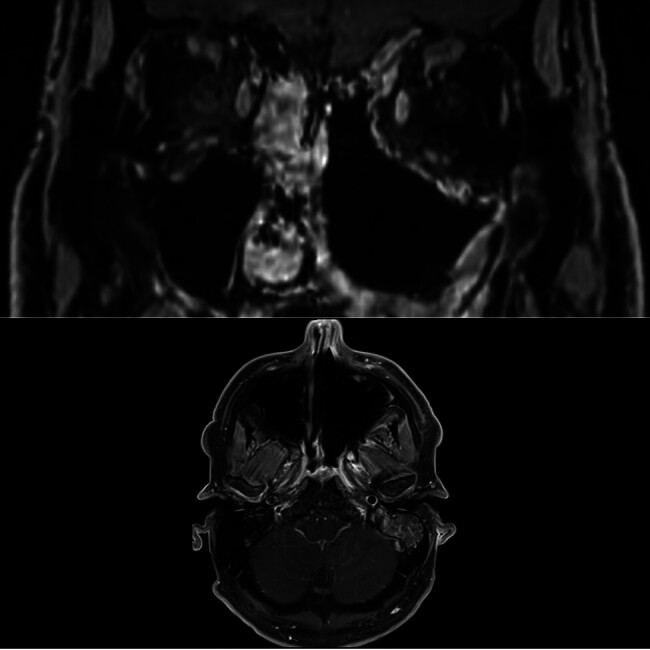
Postsurgical resection and adjuvant chemoradiotherapy, magnetic resonance imaging (MRI) of left HPV-related multiphenotypic carcinoma (HMSC) without evidence of residual or recurrent tumor.
